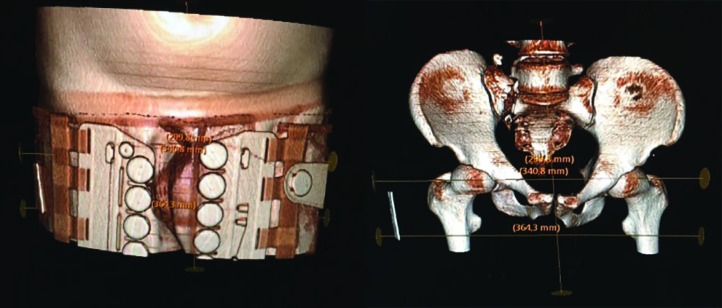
3D-CT images of a patient with the pelvic binder placed correctly – symmetrical and covering both greater trochanters
